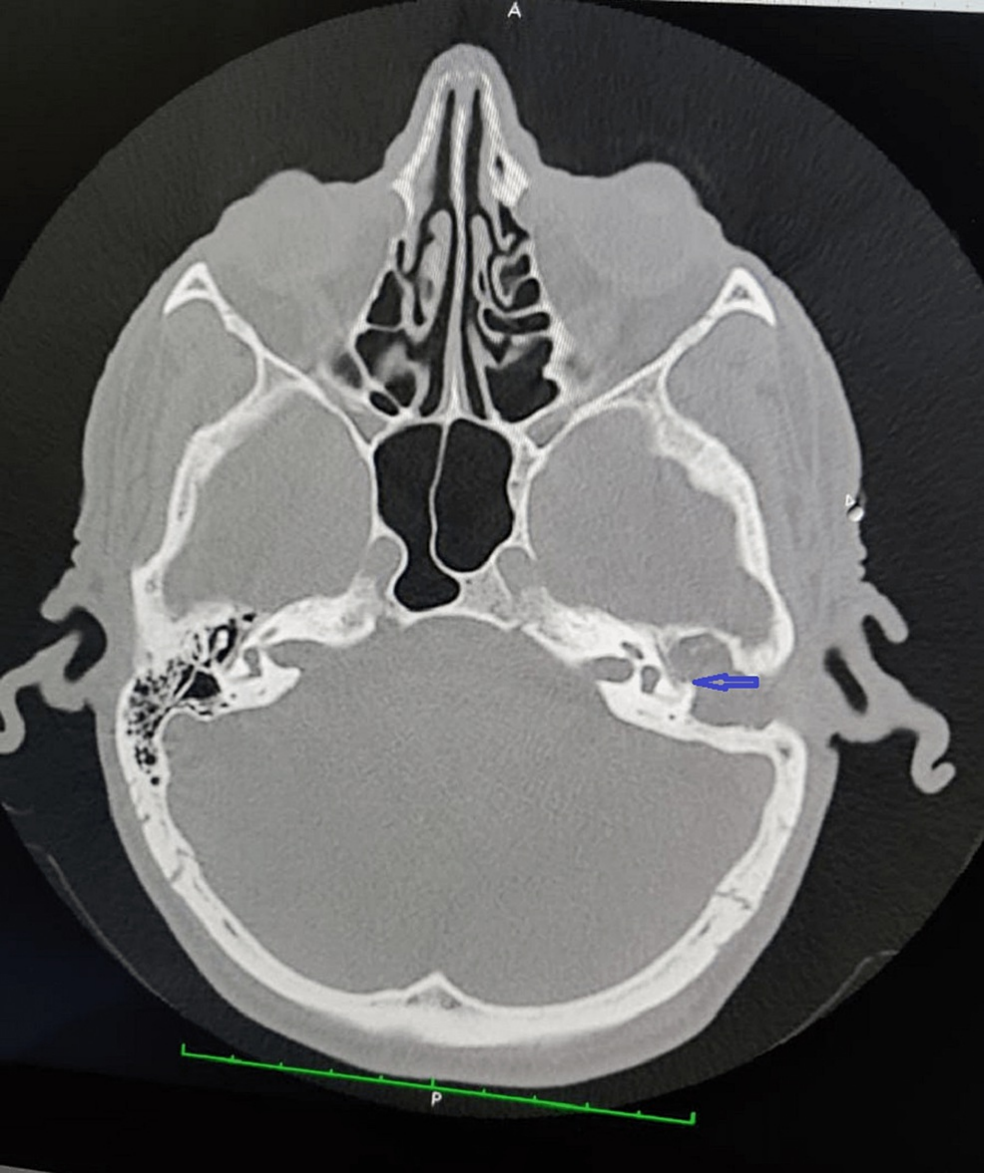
High-resolution computed tomography scan image Figure 1 depicts the axial section of a high-resolution computed tomography scan showing a type II labyrinthine fistula of the lateral semicircular canal (blue arrow).
